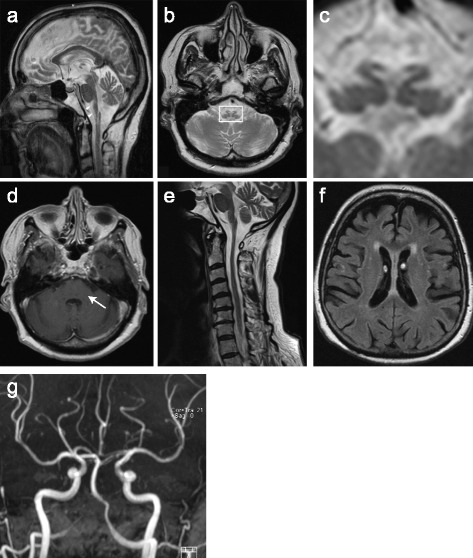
Brain stem degeneration presents in brain MRI. Sagittal (a) and transversal (b) T2-weighted brain MRI indicates gliotic neurodegeneration in the medulla oblongata with predominant loss of pyramidal tracts. Magnification of inlay (c) uncovers pathologic brain stem formation reminiscent of ‘kissing swans’. d Transversal T1-weighted sectioning of the brain stem (arrow) after Gadolinium administration. No contrast enhancement is detectable. e Sagittal T2-weighted cervical spine MRI shows atrophy of the upper cervical spinal cord in addition to medulla oblongata atrophy. f Transversal T2-weighted brain MRI indicates putative periventricular rim-sign and global brain atrophy. g Brain MRI-angiography reveals normal intracranial vascular status without indication of stenosis
